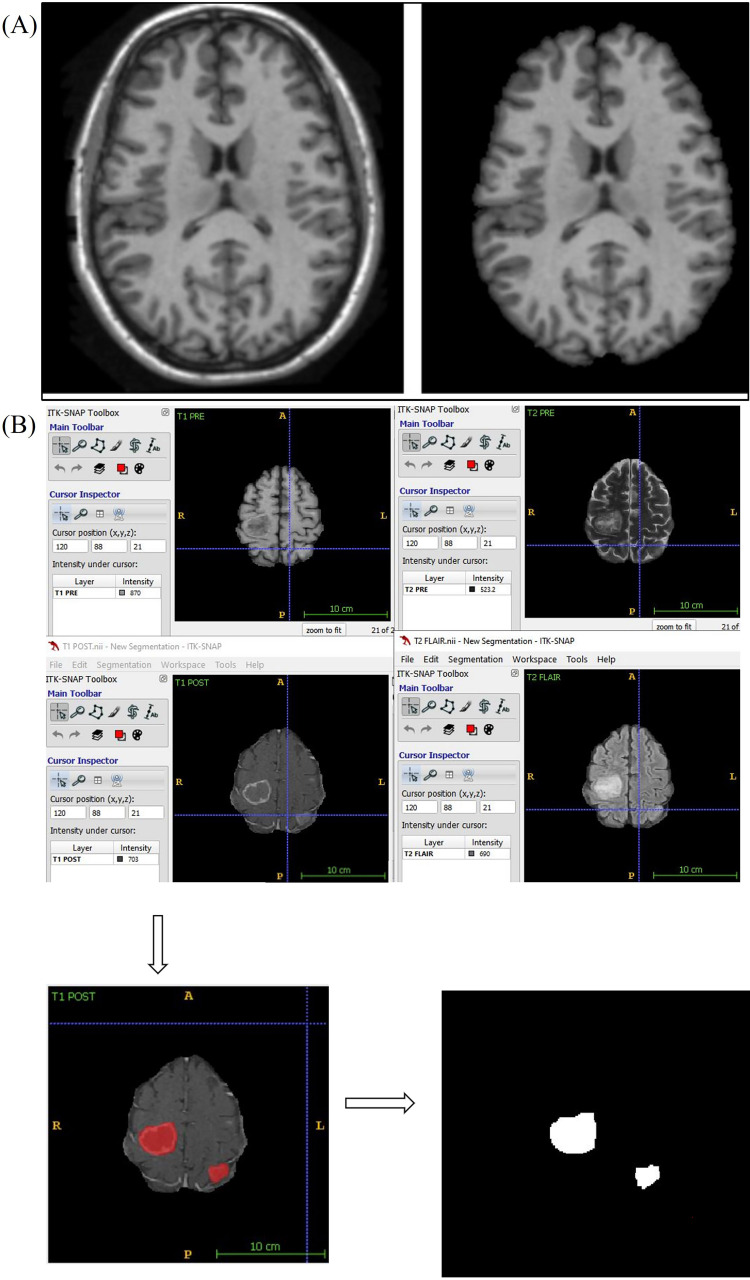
Skull stripping and annotation of images. (a) Axial T1W MRI sequence image the brain before and after skull stripping; (b) annotation of MR images. A: anterior; P: posterior; R: right; L: Left
